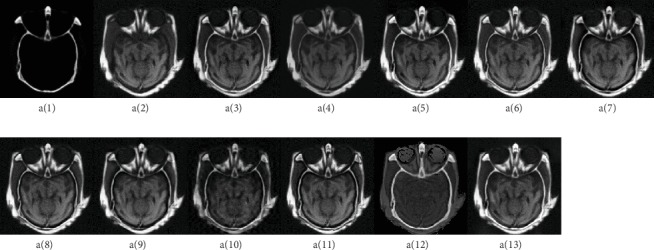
Examples of MRI-CT medical image fusion, a(1) and a(2) are CT and MRI source images, respectively. a(3)~a(13) are the fused images of GFF [81], MSA [82], NSCT+SR [83], NSCT+PCNN [84], NSCT+LE [85], NSCT+RPCNN [86], NSST+PAPCNN [26], DWT [87], DWT+WA [88], U-Net [65], and CNN [57], respectively.
